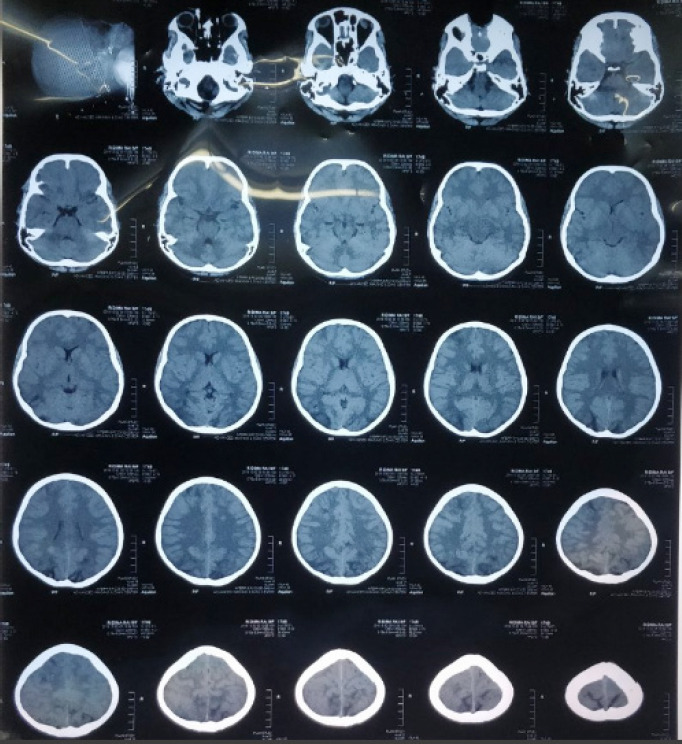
CT of the brain showing an ill-defined hypodense lesion in the bilateral occipital region.
